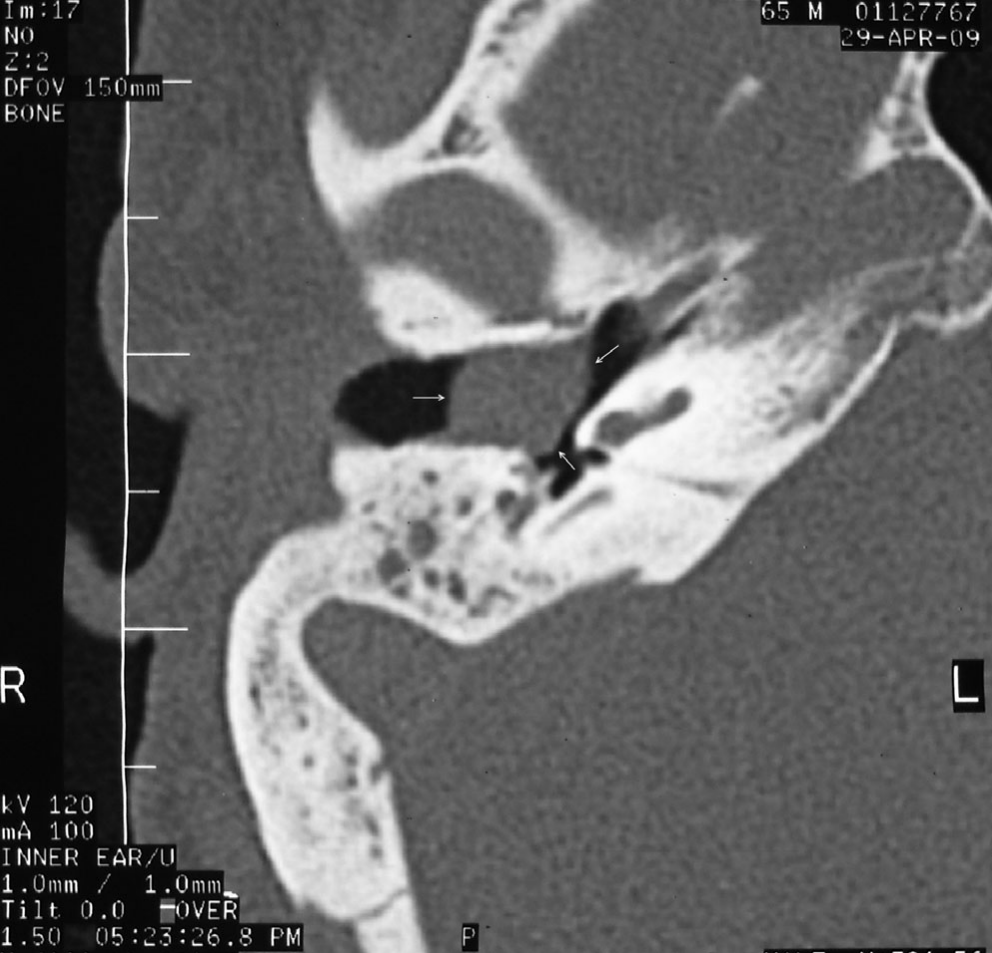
Temporal bone CT (axial) showing a soft tissue mass occupying part of the external ear canal. Arrows indicate the mass into the external ear canal.
